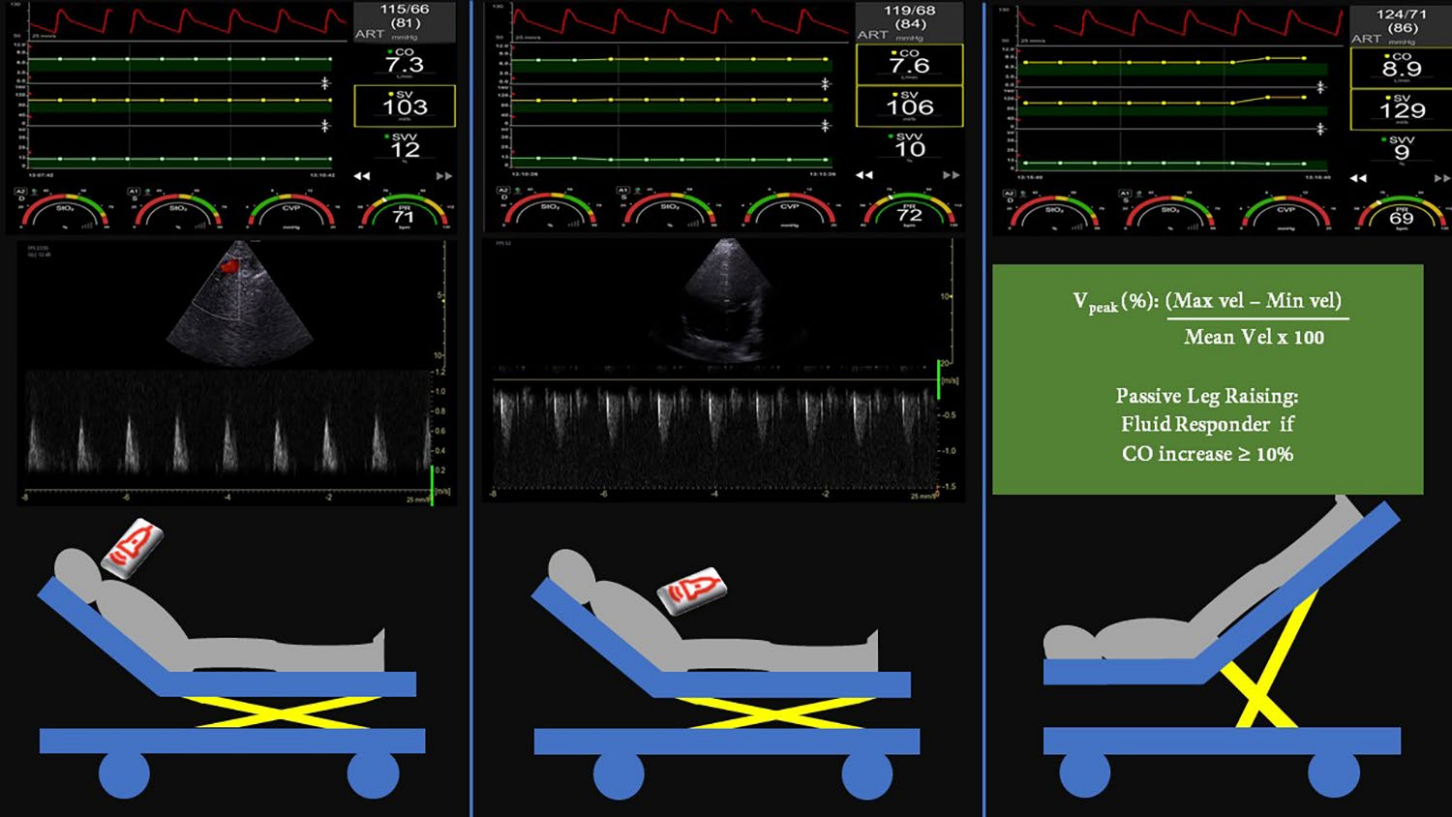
Positioning of the healthy volunteer and measurements. A and B: Semi-recumbent position used during the acquisition of images from the suprasternal A and the apical B window to obtain the respiratory variation of the peak velocity of blood flow (V_peak_). During the echocardiographic measurement, hemodynamic conditions were recorded from a finger-cuff non-invasive continuous cardiac output (CO) monitoring device. C: Participant’s position is changed with a passive leg raising maneuver, the hemodynamic changes recorded and later compared to the baseline values. ART: arterial pressure values; *PR* pulse rate (heart rate), *SV*: stroke volume, *SVV* SV variation
